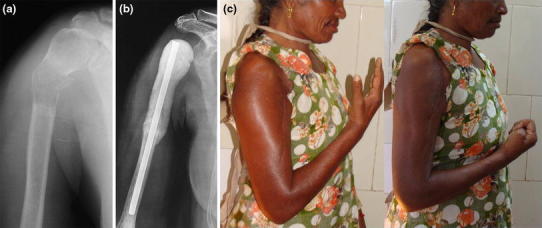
a Metastasis from thyroid preoperative X-ray. b Postoperative X-ray showing the Kuntscher’s nail cement spacer. c Good flexion of elbow and hand movements
